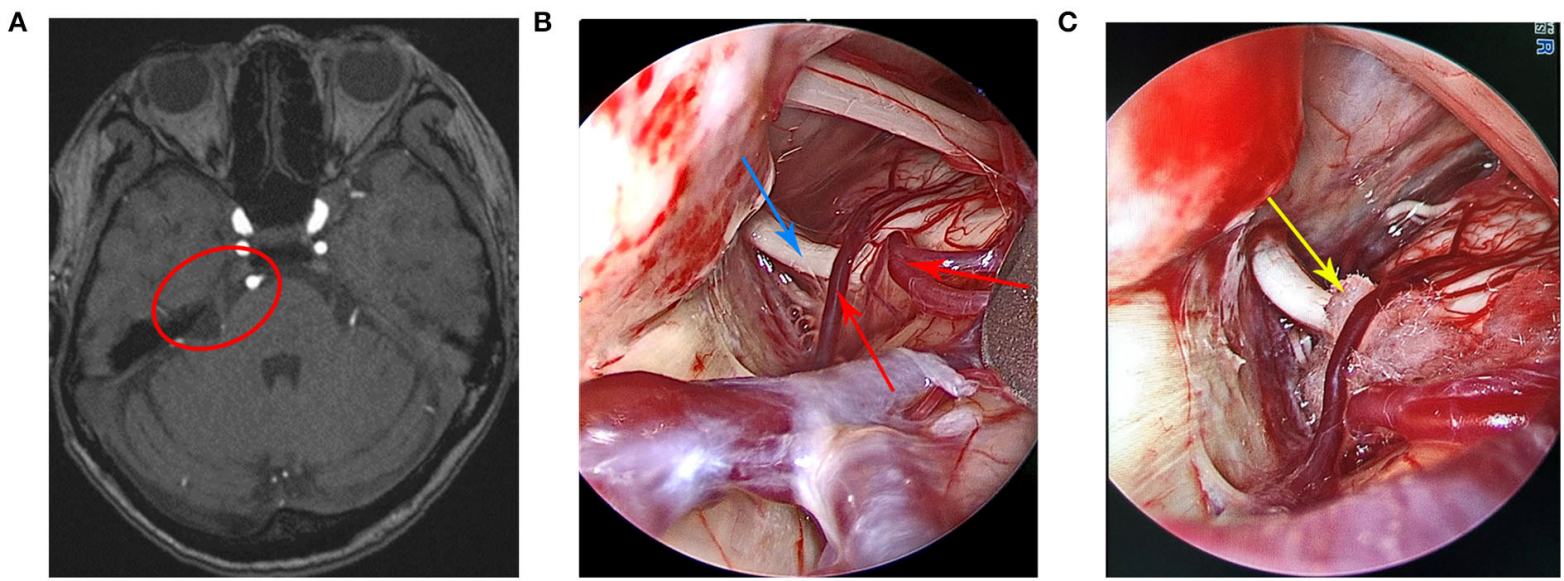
(A) Preoperative head magnetic resonance imaging of the patient. (B) A small artery, a vein that spanned the right trigeminal nerve, and the trigeminal nerve were clearly visible under the endoscopic view. (C) The Teflon pad was placed between the trigeminal nerve and the offending vessels under the endoscopic view. The red circle indicates trigeminal nerve compression by the offending vessels, the red arrow indicates the offending vessels, the blue arrow indicates the trigeminal nerve, and the yellow arrow indicates the Teflon pad.
